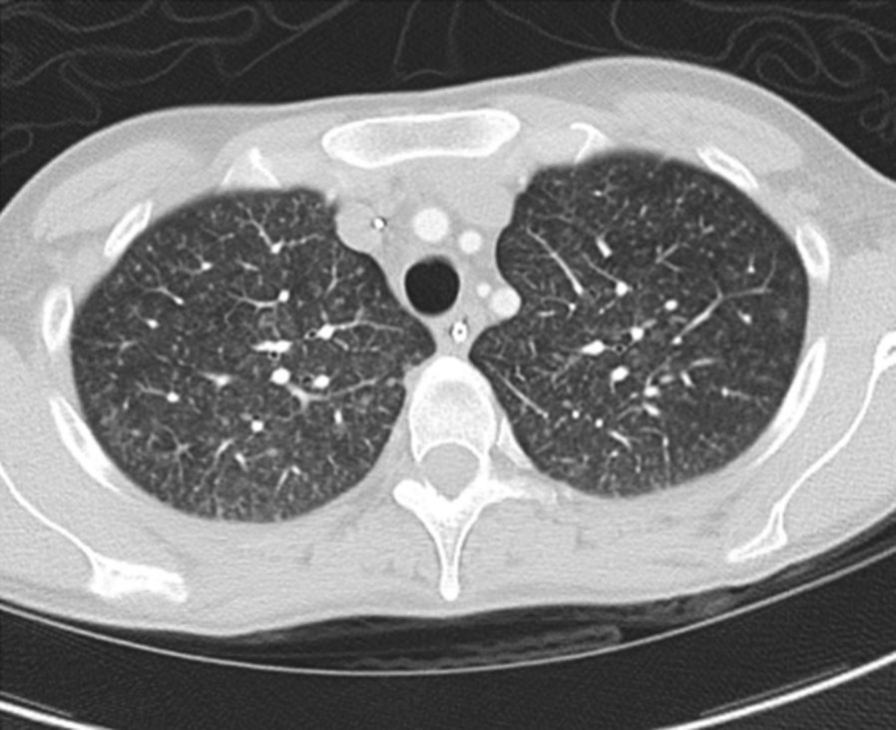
High resolution chest computed tomography scan. Centrilobular nodules in the tree-in-bud configuration.
